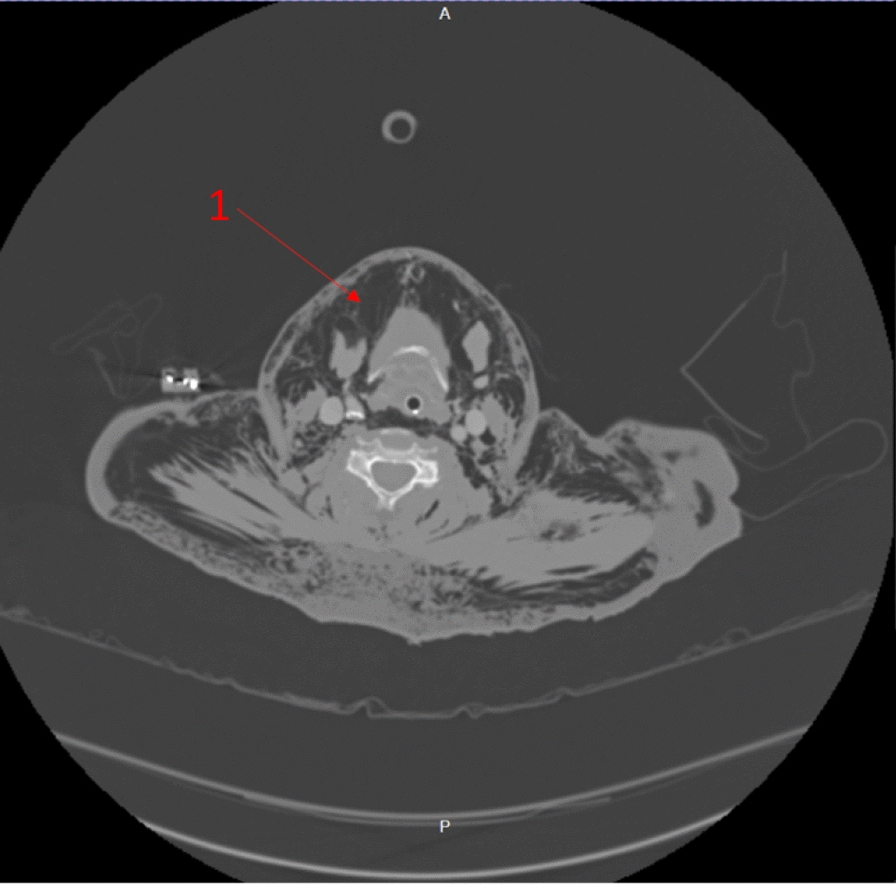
Axial computed tomography showing emphysema surrounding the larynx and laryngeal edema (arrow 1)
